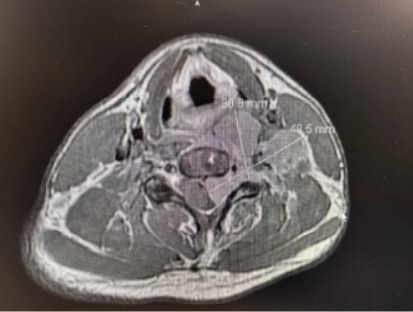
Transverse magnetic resonance imaging of the cervical spine with and without gadolinium showing dimensions of Ewing sarcoma lesion.
